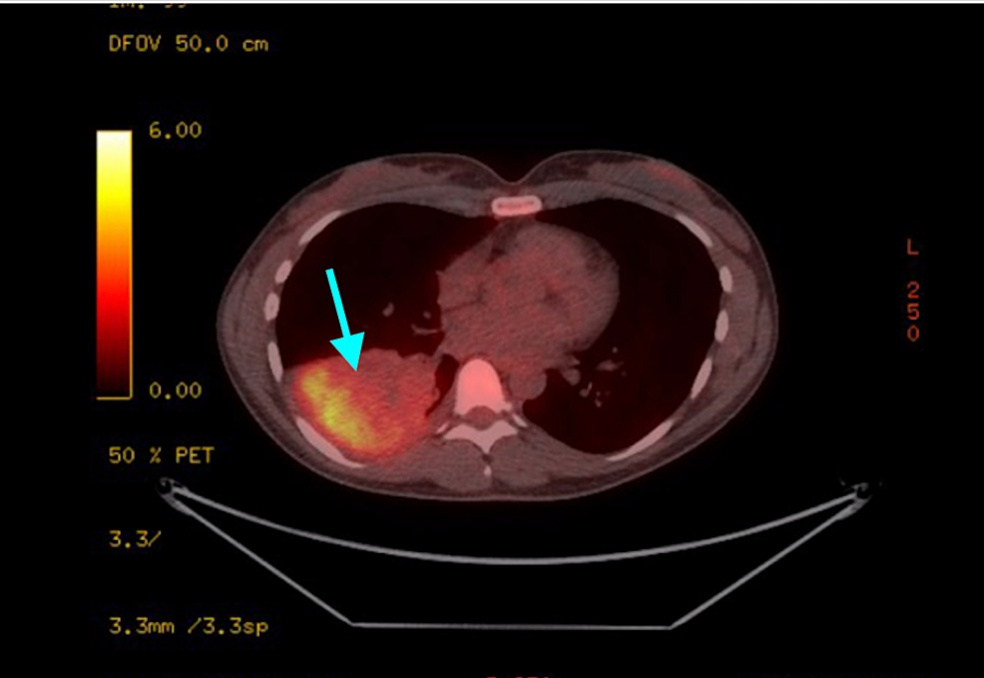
PET/CT imaging showing increased uptake in the right lower lobe (blue arrow) PET: positron emission tomography
